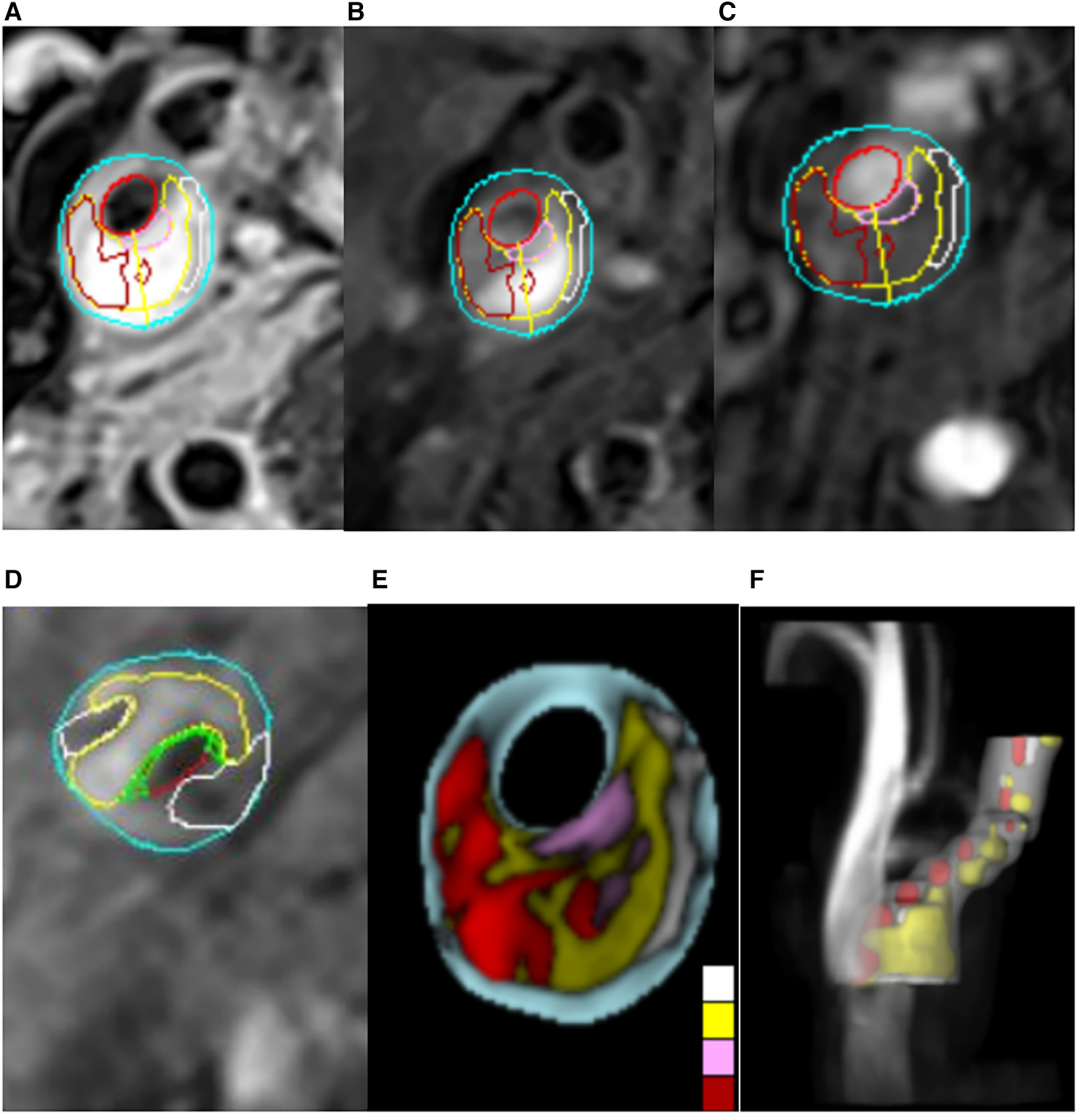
Carotid MRI semi-automatic segmentation of plaque composition. (A–D) The MRI-PlqueView vascular plaque imaging Diagnostic System was used to delineate the plaque component regions on T1-weighted imaging (T1WI), T2-weighted imaging (T2WI) and time-of-flight (TOF) sequences. Different colors indicated different plaque components. Yellow represents lipids, white represents calcification, green represents fibrous caps, dark red represents intraplastic hemorrhage, positive red represents lumen, and blue represents wall. (E,F) The MRI-PlaqueView vascular plaque imaging Diagnostic System was used to automatically recompose the cross section and 3D blood vessel images.
